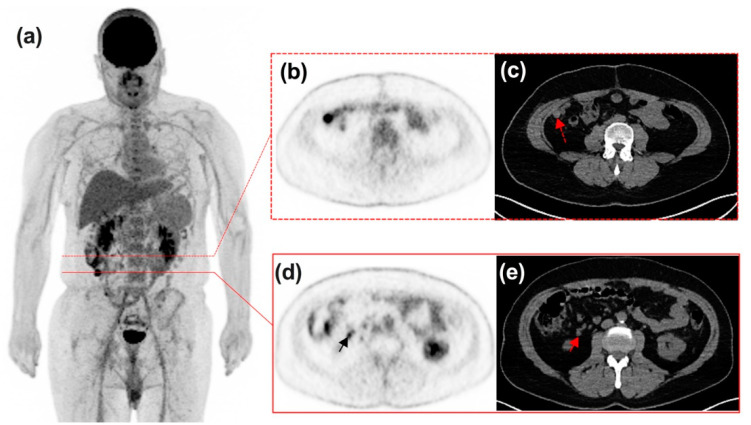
50-year-old male patient diagnosed with stage IV melanoma of lower limbs (Breslow 0.6 mm, BRAF+) and treated with TKI. ^18^F-FDG PET/CT—((a) maximum intensity view; (b,d) PET transverse slice; (c,e) CT transverse slice) depicted progression of carcinoma nodules after 19 months of treatment (red dotted arrow, red and black arrows), while ceCT determined stable disease. This patient was switched to nivolumab.
